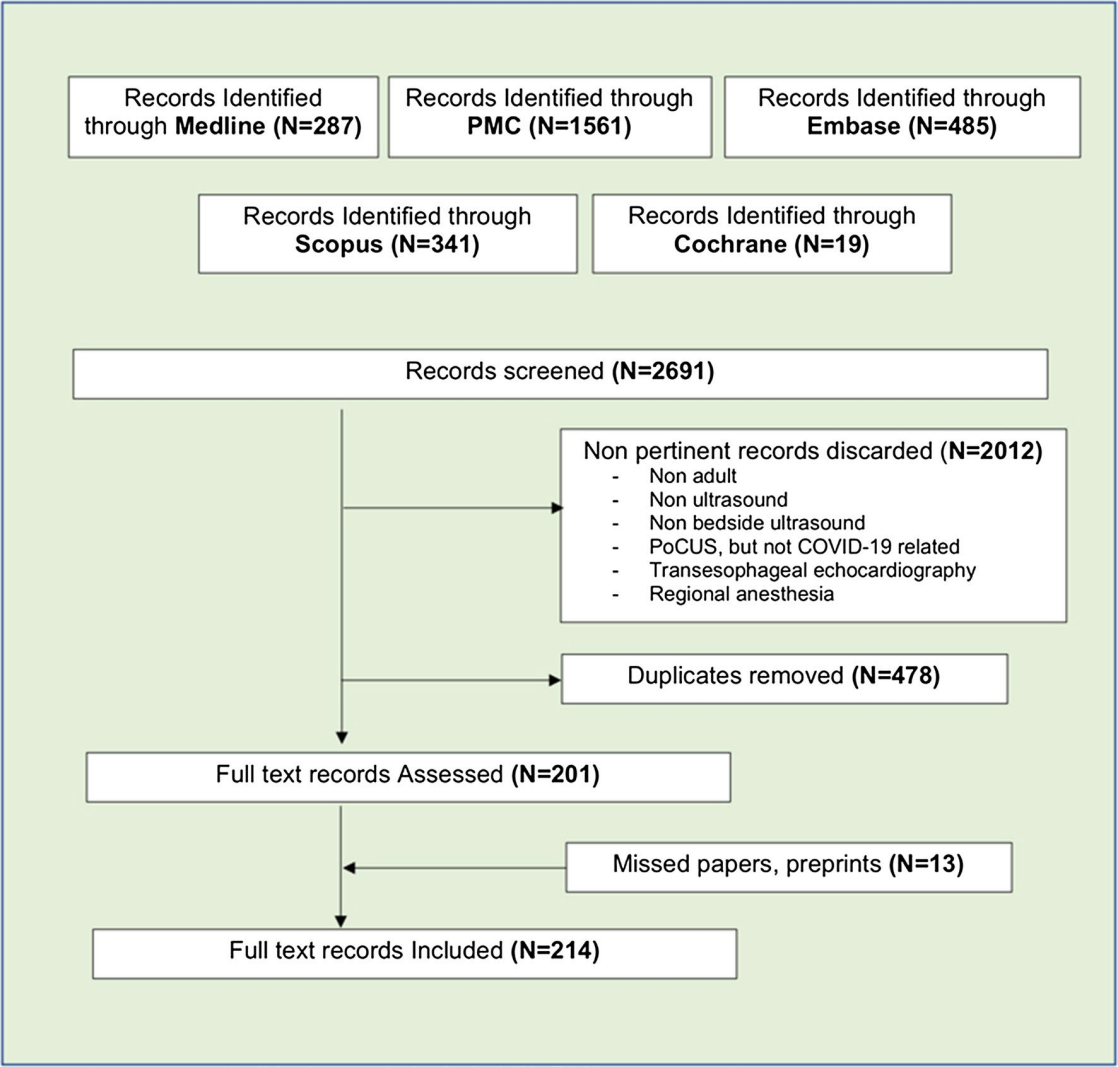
Literature search strategy. A literature search of Pubmed, Pubmed Central, Embase, Scopus and Cochrane library databases was conducted by 2 independent researchers from 01/01/2020–01/08/2020 to identify all publications on point-of-care ultrasound in COVID-19 adult patients, using English language restriction, and the following MeSH query: ((“lung” AND “ultrasound”) OR “echocardiography” OR “Focused cardiac ultrasound” OR “point-of-care ultrasound” OR “venous ultrasound”) AND (“COVID-19” OR “SARS-CoV2”). Non-pertinent findings were discarded. The references of relevant papers were hand-searched for missed papers. Duplicates were removed. An additional search of pre-print publications was made through ResearchGate, preprint online repositories and social medias
